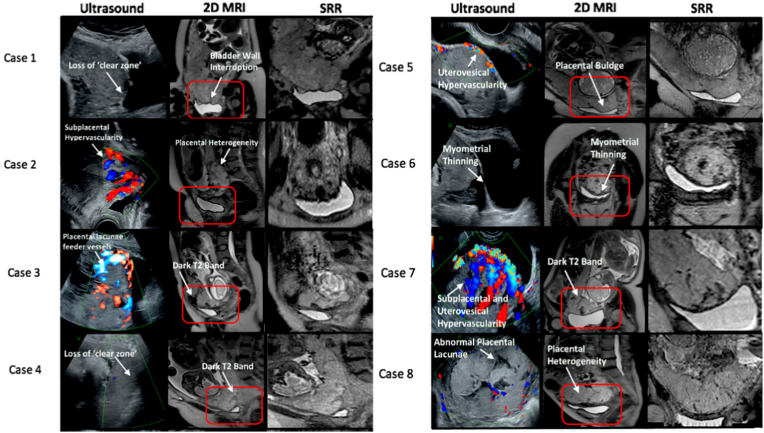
Ultrasound and magnetic resonance images of the 8 confirmed PAS cases. The first column shows ultrasound images. The box indicates the placenta-myometrial-bladder interface on the 2-dimensional (2D) MRI image (second column) that was reconstructed (third column, SRR). The arrows and labels indicate examples of pathological ultrasound and MRI markers in PAS disorder. 2D, 2-dimensional; MRI, Magnetic Resonance Imaging; SRR, Super Resolution Reconstruction.
